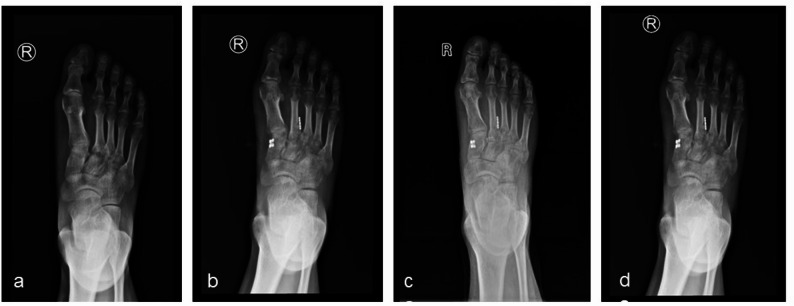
Patient A, a 36-year-old male. a Preoperative weight-bearing anteroposterior radiograph shows significant widening of the gap between the medial cuneiform and the second metatarsal. b, c, d Postoperative weight-bearing anteroposterior radiographs at 1, 3, and 6 months show good reduction of the second metatarsal, satisfactory position of internal fixation without loosening, breakage, or displacement, and no narrowing or degenerative changes in the tarsometatarsal joints
